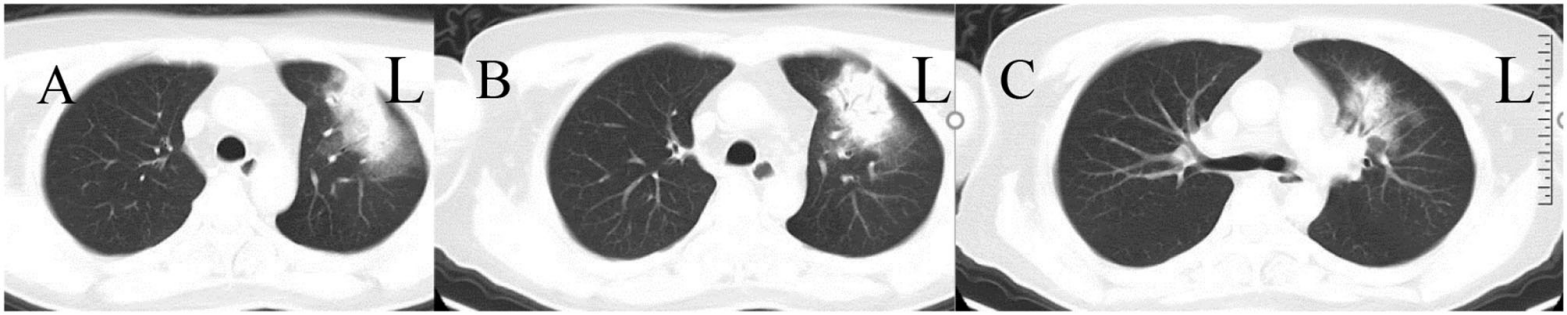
CT scan of the patient (A–C show CT scans on the 0th day after admission).
